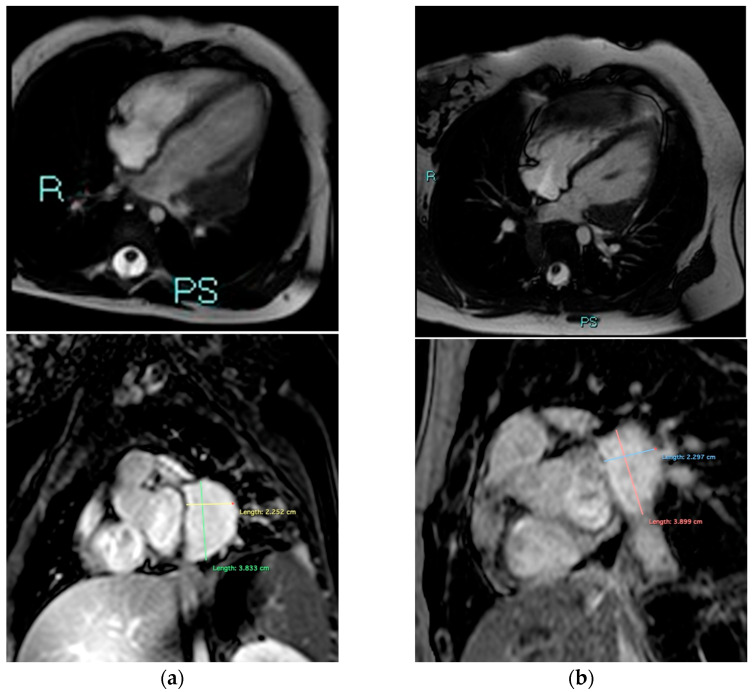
Cardiac MRI of the patient, performed at the onset of the cardiological symptoms (a) and after an 11-year-long follow-up (b), showing the stability of the size of the cardiac fibroma (main axis measures are shown in millimeters). (Upper) images: 4-chamber cine view, acquired with Steady-State Free Precession (SSFP) sequence. (Lower) images: Phase-Sensitive Inversion Recovery (PSIR) sequence on short axis plane, with a T1-weight (TI) of 320 ms (MRI scanner Philips 1.5T).
